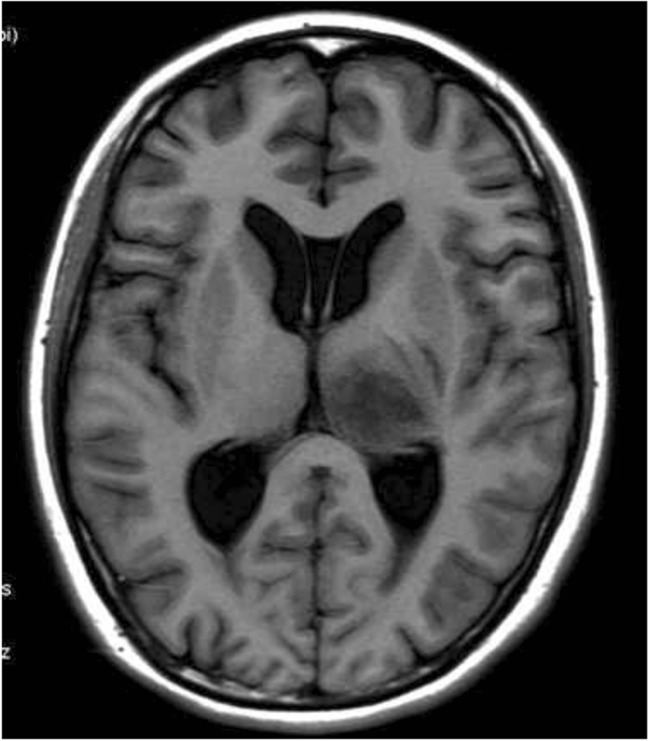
Axial cranial MRI localization slice at the level of the basal ganglia
